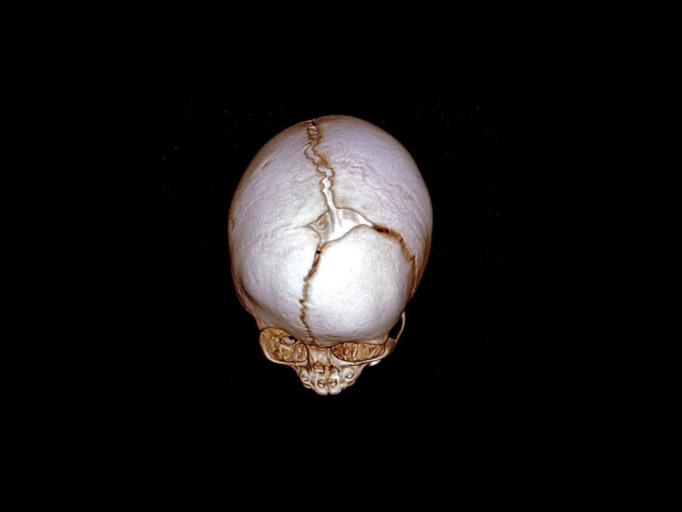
Computed tomography 3D images of the skull of the proband from Family 2, showing the asymmetric craniosynostosis due to the precocious closure of the right coronal suture.
